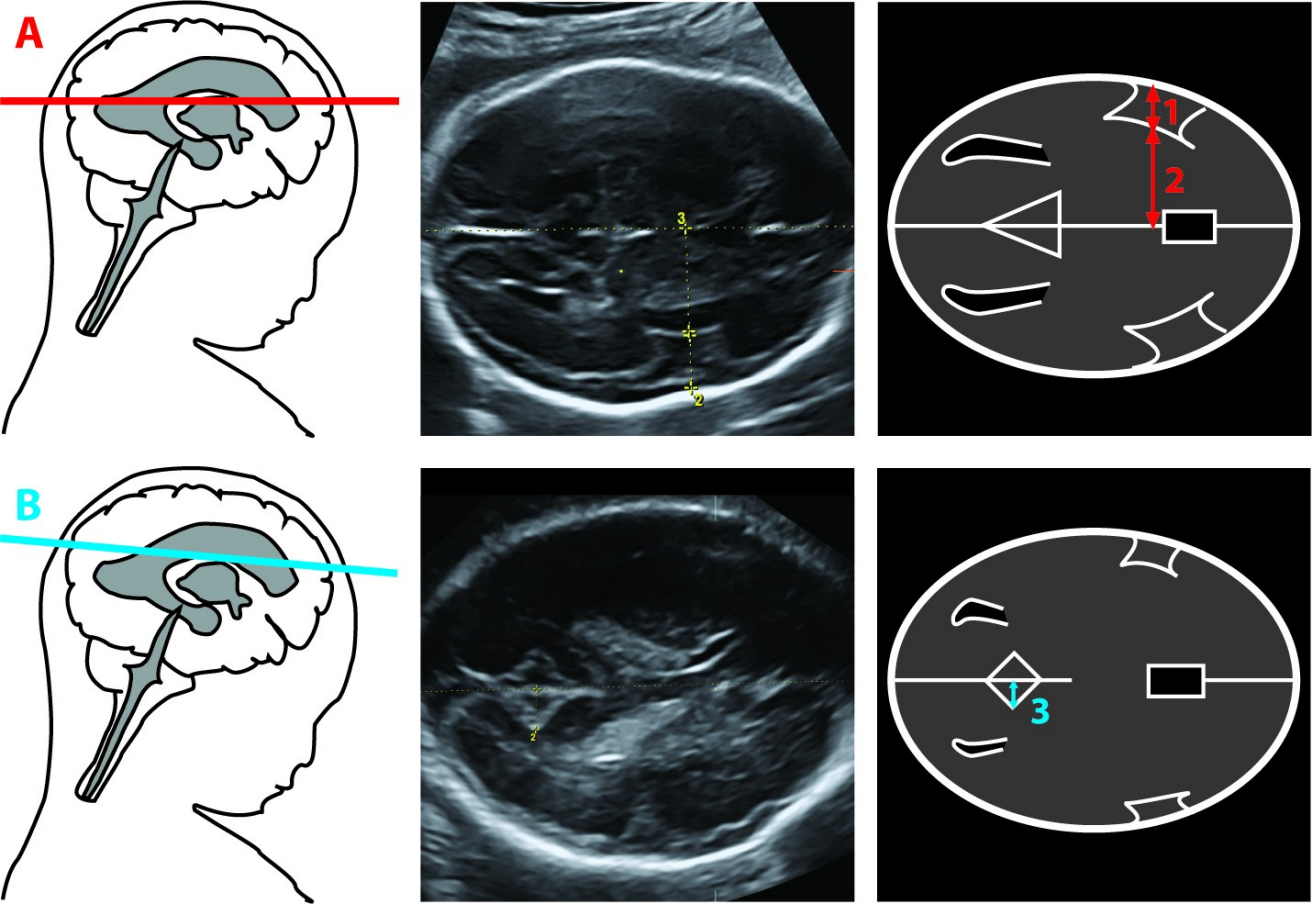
Brain fissure depth measurements using 3D-Ultrasound [6]. The measurement of the Insula depth (Fig 1A, measurement 2), the Sylvian fissure (Fig 1A, measurement 1) and The POF (Fig 1B, measurement 3).
